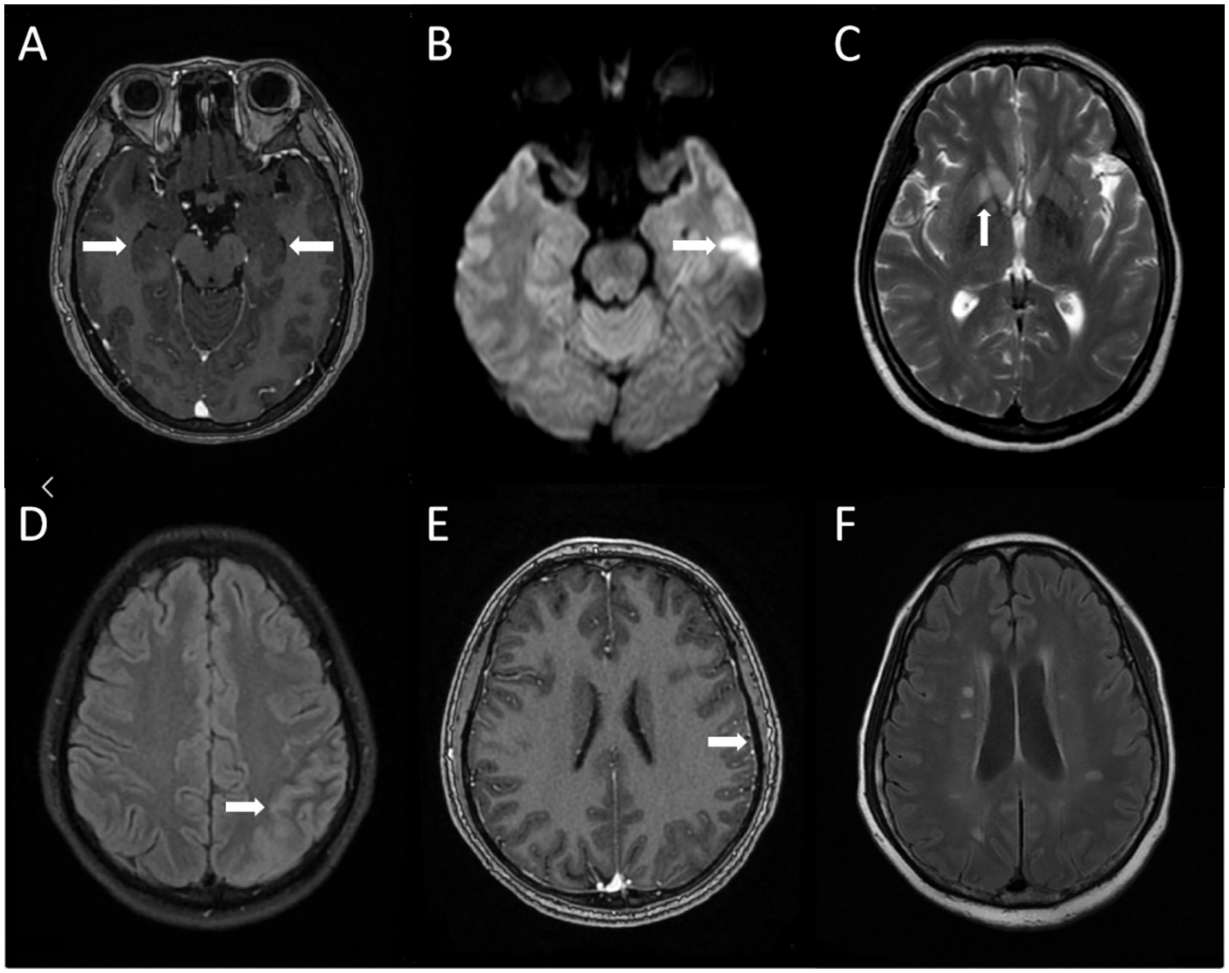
Brain MRI Characteristics of AE Patients. (A) Brain magnetic resonance enhanced scan in case 9 showed bilateral hippocampal swelling with abnormal signal; (B,C) brain magnetic resonance scan in case 10 showed abnormal signal of left temporal lobe and right basal ganglia; (D,E) brain magnetic resonance plain scan in case 13 showed the left temporal parietal lobe gyrus is swollen and the cerebral magnetic resonance enhanced scan in case 13 showed adjacent meninges are slightly thickened; (F) brain magnetic resonance plain scan in case 14 showed multiple abnormal signals in bilateral corona radiata.
